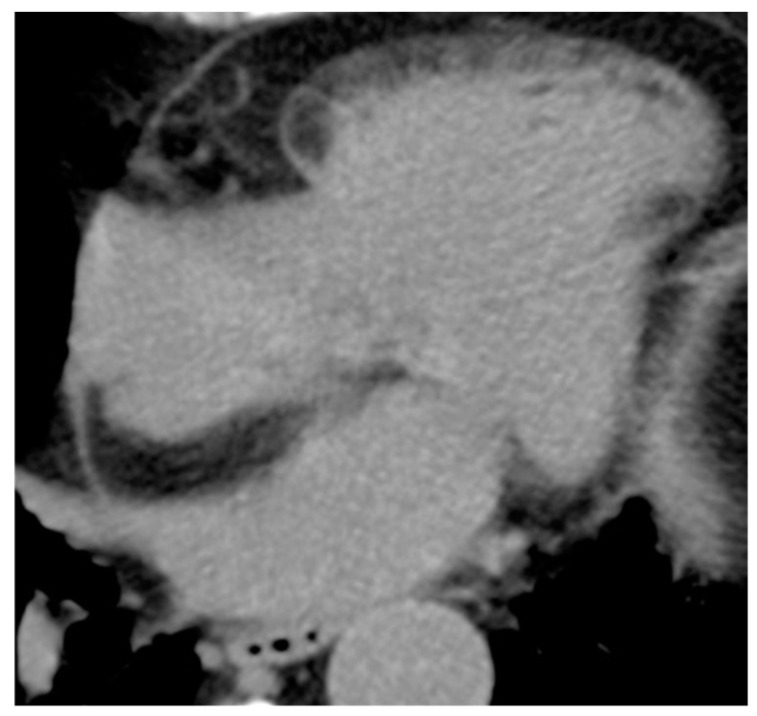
81-year-old female patient with lipomatous hypertrophy of the interatrial septum in CT scan.
